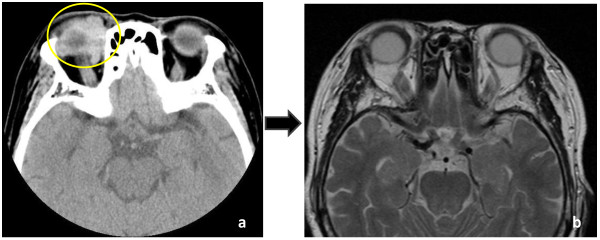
Patient presented with swelling and ptosis of the right upper eyelid. (a) Head CT of the orbit revealed abnormal high density area (yellow circle); (b) MRI revealed the presence of an infiltration of the right intraorbital diffuse mass which regressed and decreased in size one month after treatment was initiated.
